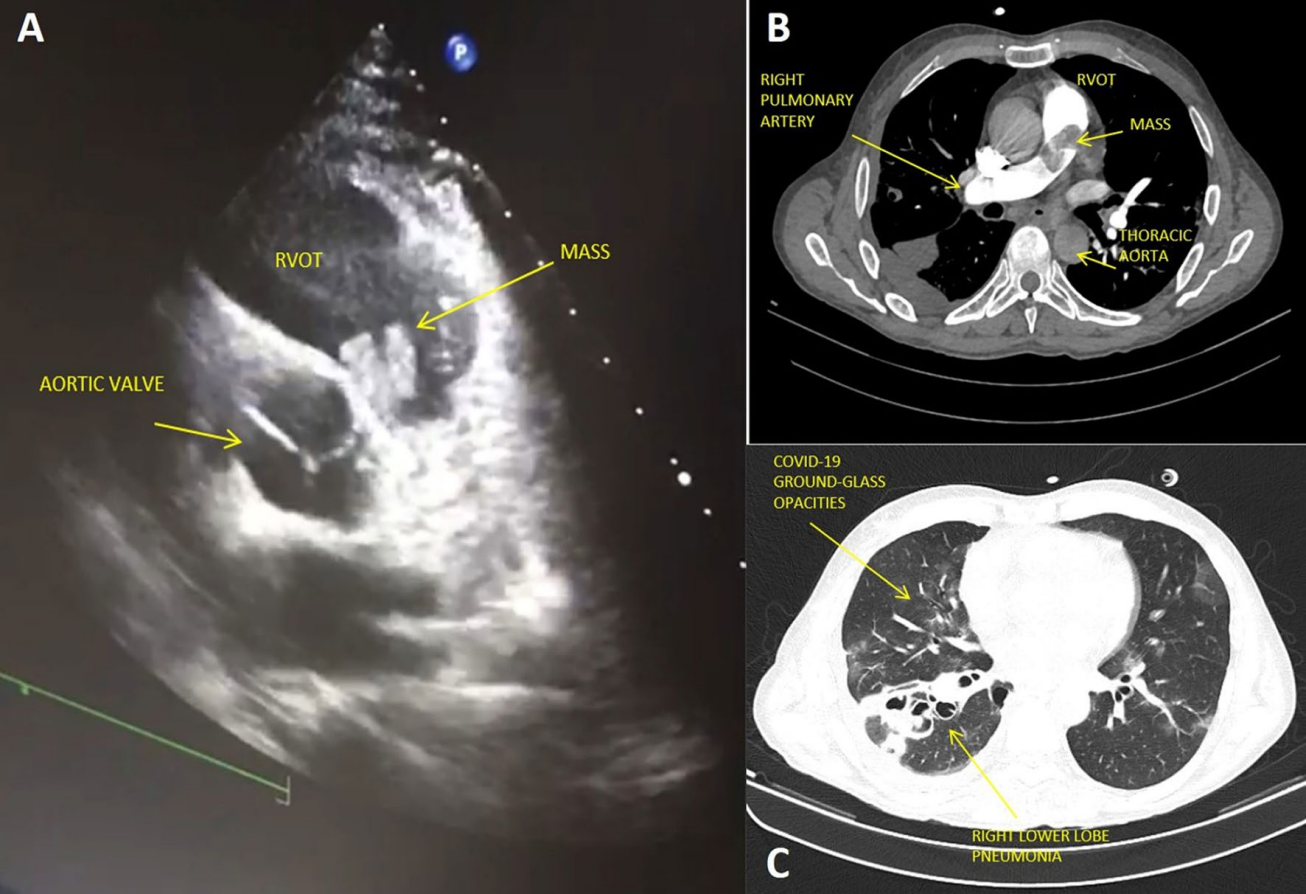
A Transthoracic echocardiogram showing PV mass protruding in the RVOT. B Chest CT scan showing PV mass extending from the RVOT to the right pulmonary artery. C Chest CT scan showing interstitial pneumonia with ground-glass opacities and right lower pneumonia with confluent abscesses
